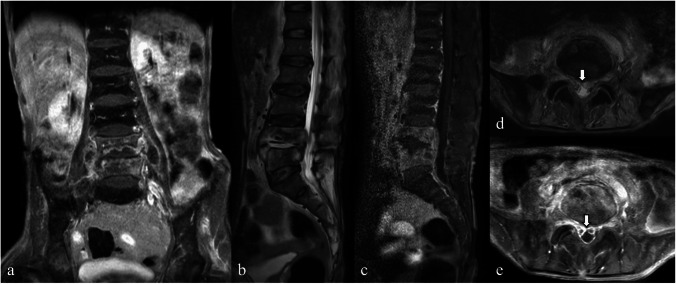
Magnetic resonance images of a 79-year-old male patient with tuberculous spondylitis. Coronal fat-suppressed contrast-enhanced T1-weighted (a), sagittal T2-weighted (b), fat-suppressed contrast-enhanced T1-weighted (c) images show tuberculous spondylodiscitis with perivertebral inflammatory soft tissue and anterior epidural abscesses. Axial T2-weighted (d) and fat-suppressed contrast-enhanced T1-weighted (e) images reveal preservation of the anterior meningovertebral ligament resulting in a bilobed appearance of anterior epidural abscess (arrows)
